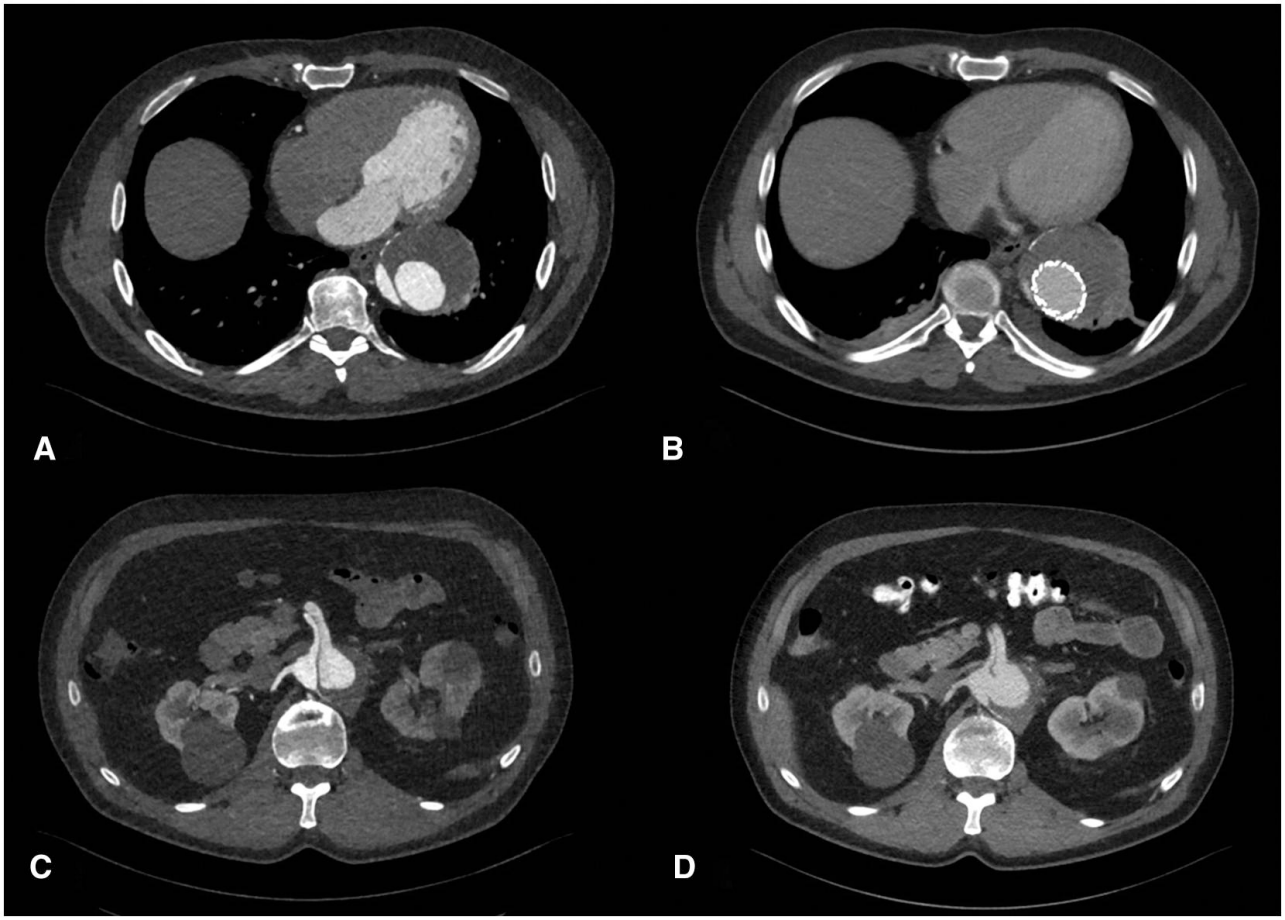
Axial Contrast-Enhanced CT Images before (2A, C) and after (2 B, D) EASE and TEVAR. Thoracic Aortic Diameter up to 6.8 × 7.5 cm; Abdominal Aortic Diameter up to 5.4 × 4.9 cm.
